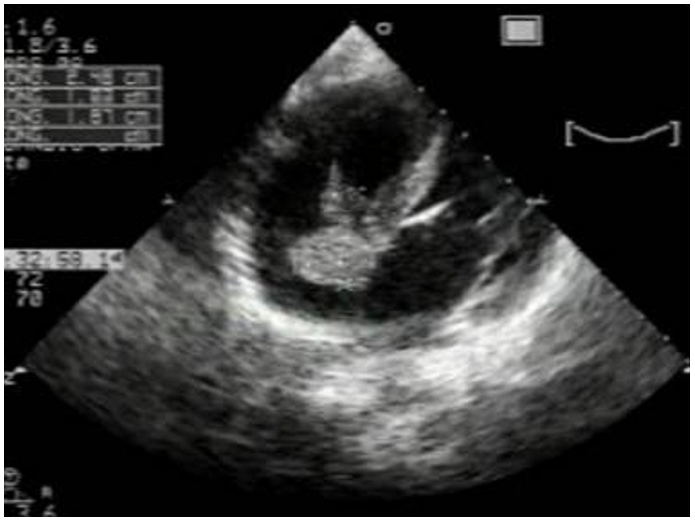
Two-dimensional echocardiography, in the apical four chamber view showing a large interatial septum cystic mass in a 16 years old Tunisian with cardiac hydatid cyst
